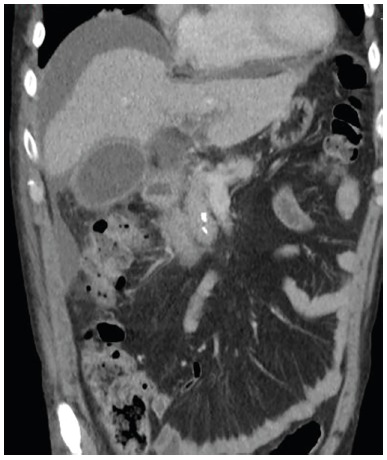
Preoperative abdominal CT scan: free fluid can be seen in the right
